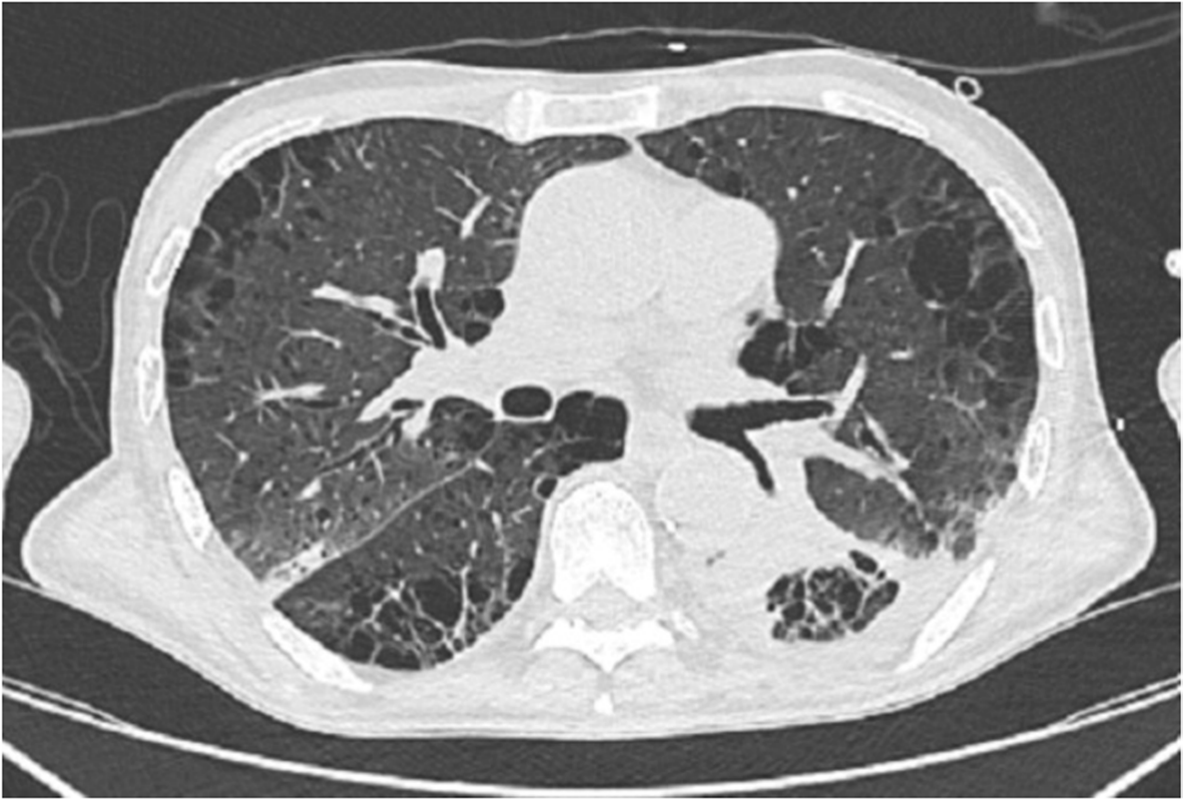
Pattern of interstitial pneumonia on chest CT scan
